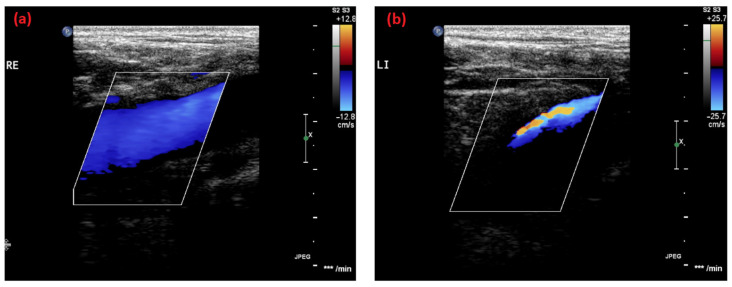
Ultrasound images of the (a) right and (b) left femoral vein of the 19-year-old brother. Turbulence and a decrease in vein diameter in the left vein compared to the right side indicate a venous compression syndrome.
